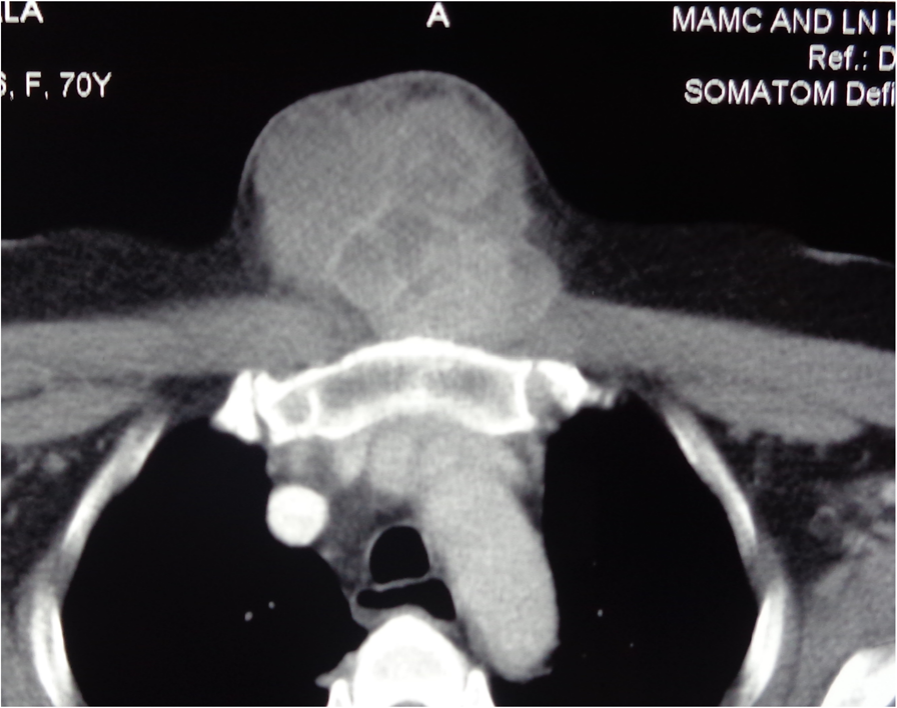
CT scan showing soft tissue mass anterior to sternum
